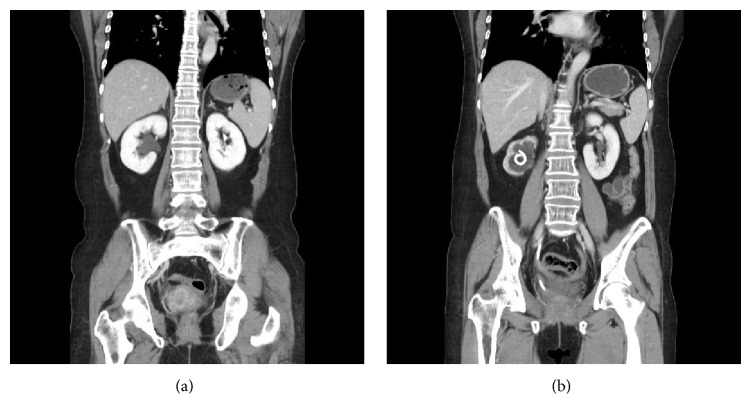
A CT scan of a patient with right hydronephrosis (a). Prominent shrinkage of renal parenchyma was noted (b) after 13 months of ureteral stent placement.
